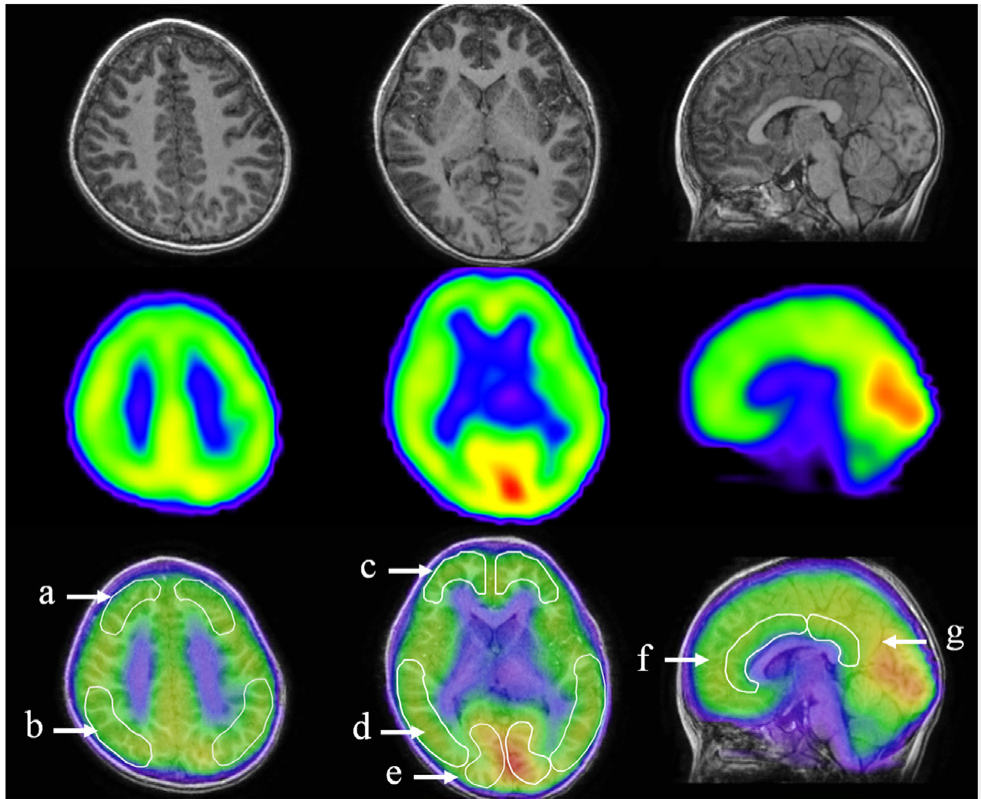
Designated regions of interest (ROIs) in fusion images of ^123^I-iomazenil SPECT and MRI. The top panel shows brain MRI (transverse and sagittal T_1_ sequences), the middle panel the corresponding results of ^123^I-iomazenil SPECT, and the bottom panel fusion imaging. Outlined regions in the bottom panel indicate designated ROIs, namely, (a) the superior frontal, (b) parietal, (c) frontal, (d) middle temporal, and (e) occipital regions; (f) anterior and (g) posterior cingulate gyrus.
